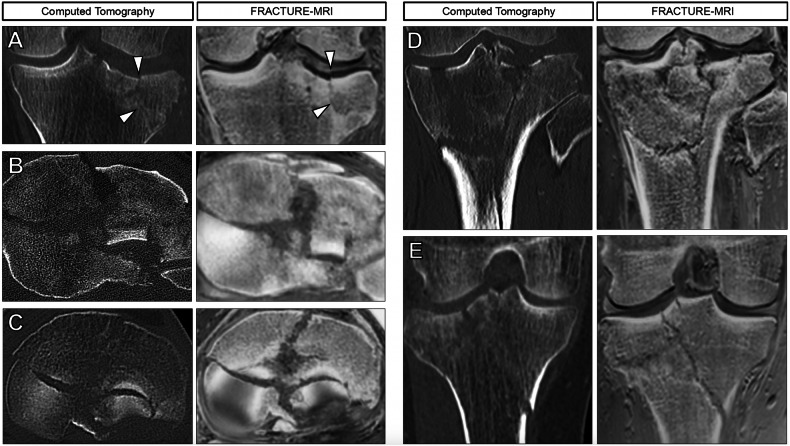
Comparison of exemplary displaced tibial plateau fractures in computed tomography (CT, left columns) and FRACTURE-MRI (right columns). Arrowheads highlight fracture lines. A Coronal images show an impression of the lateral tibial plateau with an associated fracture line in a 67-year-old female (AO/OTA B3, Schatzker II). B Axial images show displaced bicondylar fractures in a 61-year-old male (AO/OTA C3, Schatzker VI) and (C) in a 60-year-old male (AO/OTA C3, Schatzker VI). D Coronal images show displaced bicondylar fractures in a 61-year-old male (AO/OTA C3, Schatzker VI) (E) and a 25-year-old male (AO/OTA C3, Schatzker VI). Fracture lines are sharply delineated on FRACTURE-MRI
